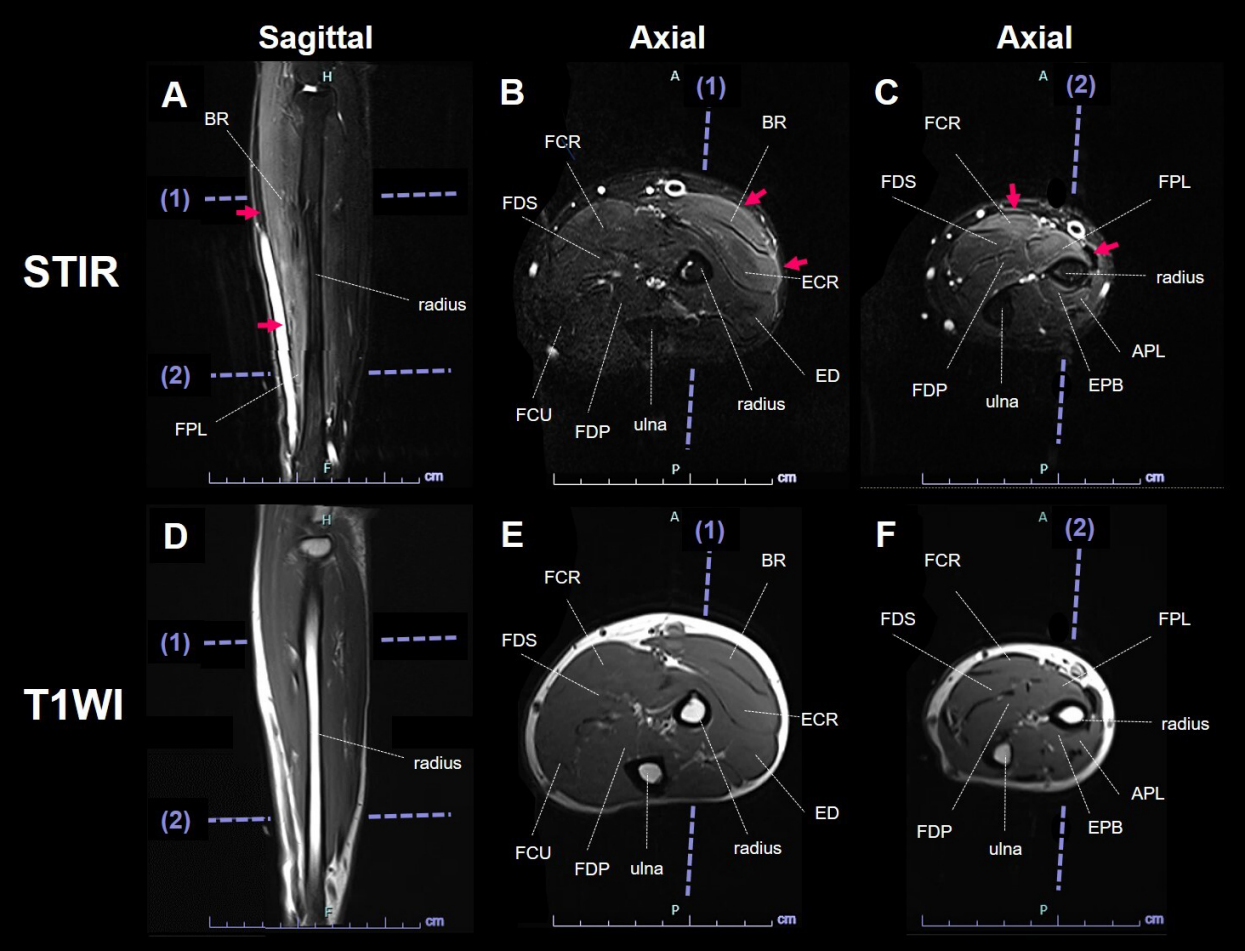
MRI of the left forearm. STIR sagittal image (A) and axial images (B, C) show hyperintensity in the muscle and fascia in the BR, ECR, FCR, and FPL muscles (A-C, arrows). The FDP muscle appeared intact. T1-weighted images show normal findings (D-F). APL: abductor pollicis longus; BR: brachioradialis; ECR: extensor carpi radialis longus and brevis; ED: extensor digitorum; EPB: extensor pollicis brevis; FCR: flexor carpi radialis; FCU: flexor carpi ulnaris; FDP: flexor digitorum profundus; FDS: flexor digitorum superficialis; FPL: flexor pollicis longus; MRI: magnetic resonance imaging; STIR: short-tau inversion recovery; TIWI: T1-weighted image.
